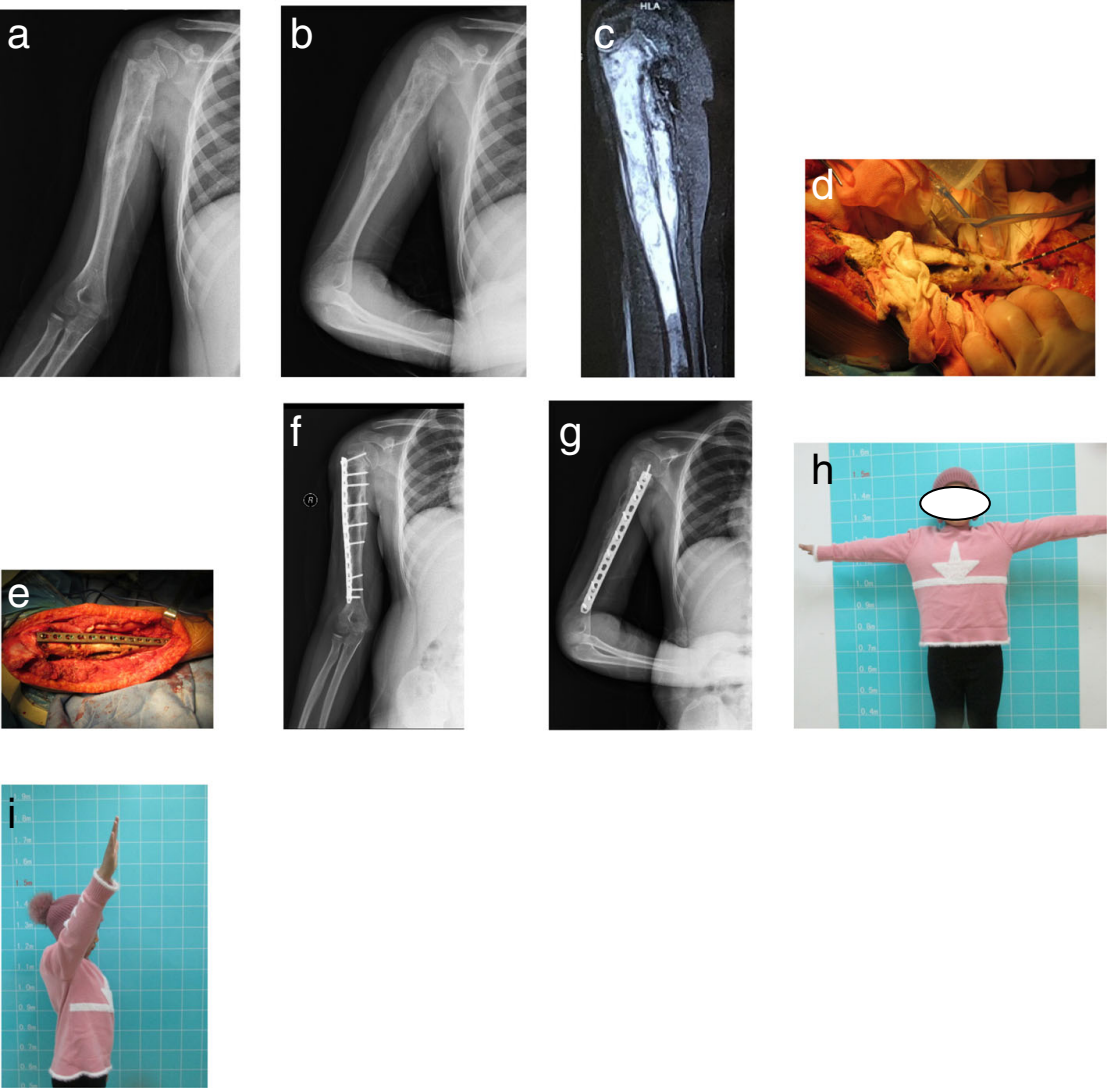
Case 7, a 8-y old female patient with Ewing sarcoma in the right humerus(S45E1). The preoperative bone strength score is 12. a, b The post-chemotherapy X-ray showed that obvious calcification after chemotherapy. c The post-chemotherapy MRI showed that tumor did not invade epiphyseal plate with a clear bounder. d, e This patient underwent in situ microwave ablation and plate internal fixation. f, g 15 months after operation,the X-ray indicated that internal fixation was in good position and no local recurrence of tumor occurred. h, i The patient received excellent abduction and lifting function of shoulder joint at the last follow-up
